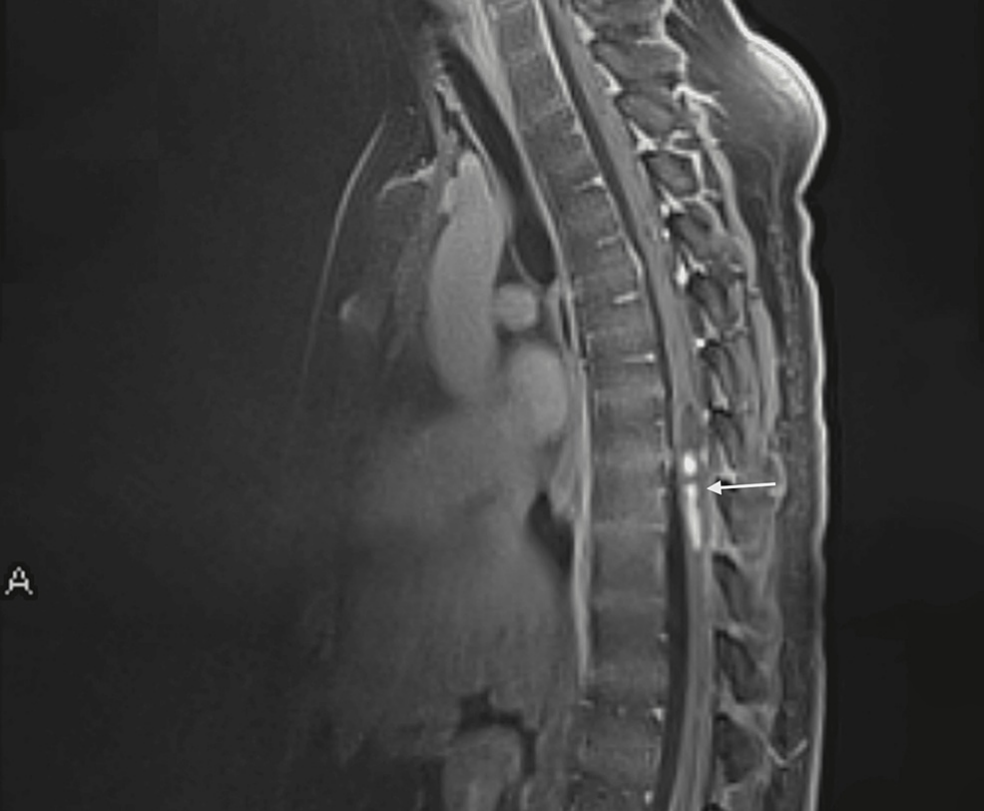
Sagittal spinal contrast-enhanced T1-weighted imaging demonstrating hyperintense enhancing lesions with some cystic segments (arrow), expanding the spinal cord, most prominently around T8-T9
